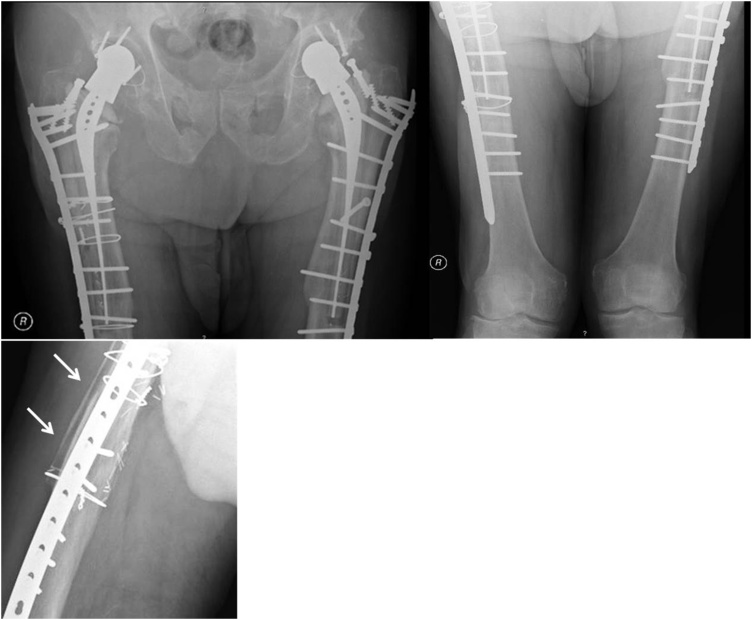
Postoperative radiograph images of the pelvis and of bilateral femur after open reduction and ORIF with locking compression plates. At follow-up period of 12 months, the X-rays showed bone union of both fractures and bilateral stable stem fixation. The lateral radiograph of the right femur showed also no signs of bone resorption of the fibular allograft.
